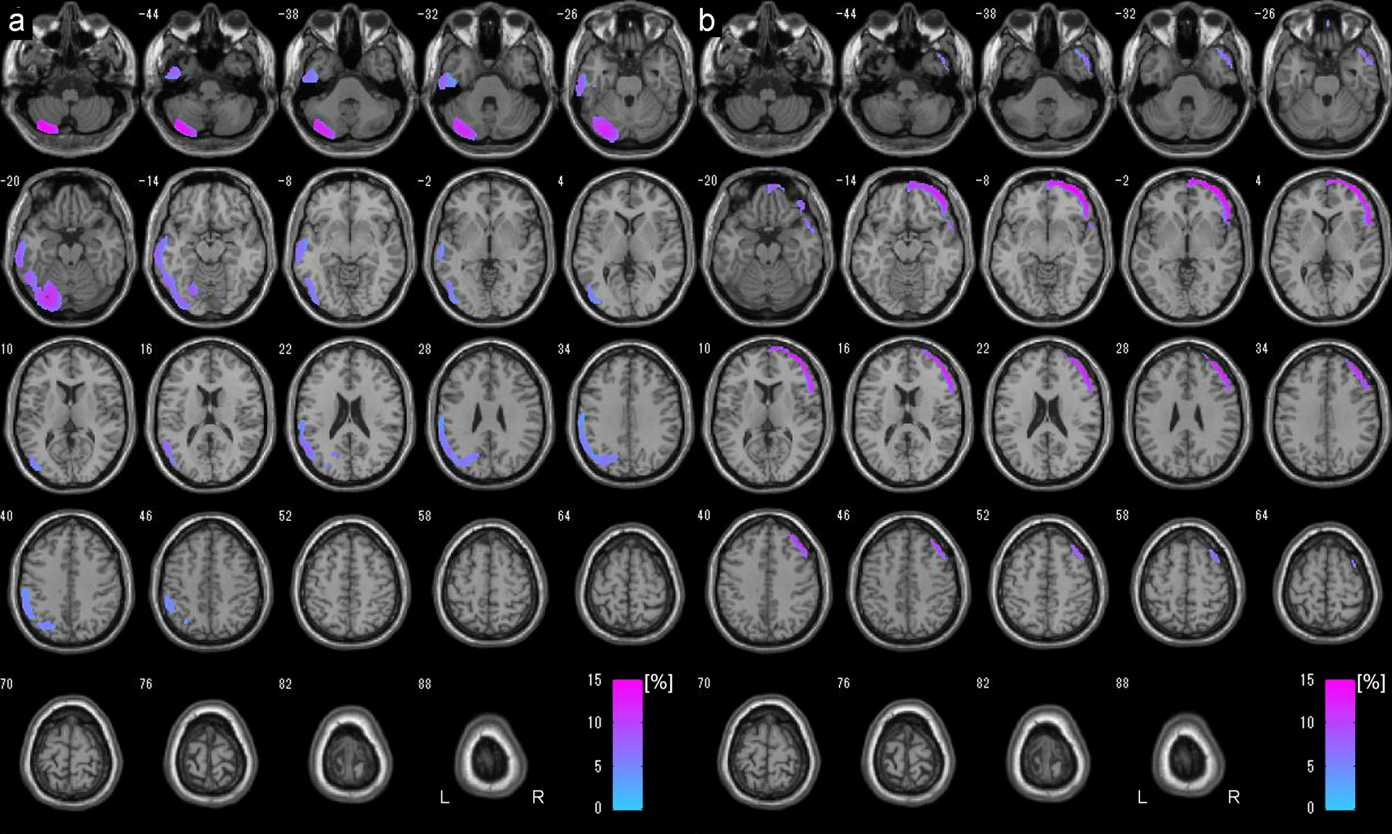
Postoperative CBF decreased areas displayed in transverse sections. Decreased ratio (%) at the area of significantly decreased CBF on postoperative SPECT compared with preoperative SPECT was shown in contralateral hemisphere to the STA-MCA bypass site by SPM8 with DARTEL (a). Since global mean normalization to the value of 100 was conducted for all SPECT data, ipsilateral CBF increase in wide area is likely to cause pseudo CBF decrease in the contralateral site. The conventional analysis showed significant decreased CBF areas on the surface of the ipsilateral hemisphere of bypass site (b). No decreased CBF areas were detected in the contralateral hemisphere, which suggests that decreased CBF areas in the operated hemisphere compensate CBF increase from the viewpoint of global mean normalization method.
